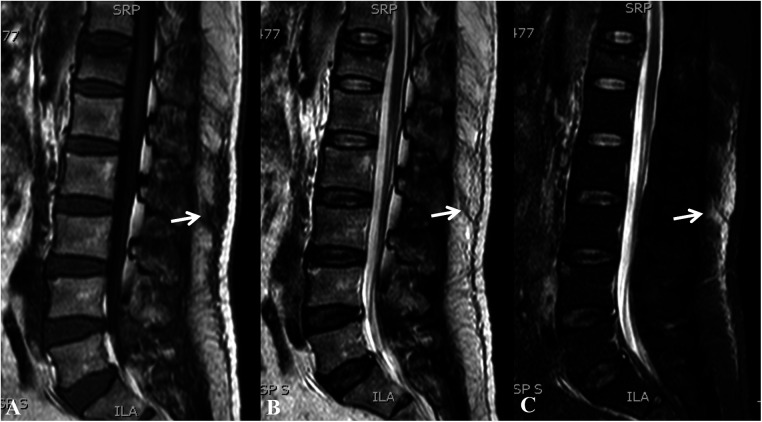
T12 vertebral compression fracture with thoracolumbar fascia injury (T12-S1) showed low signal intensity on T1 WI (A, arrow) and high signal intensity on T2 WI (B, arrow) and T2-STIR WI (C, arrow) on magnetic resonance imaging.
